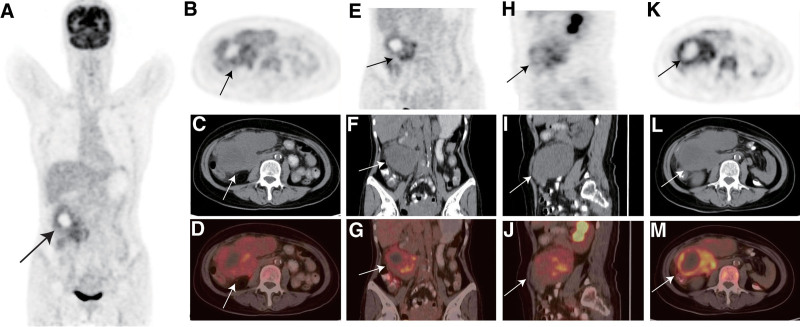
Maximum intensity projection image (A) showing a large irregular abnormal activity in the right abdomen (Black arrow). Axial (B: PET, C: CT, D: fused PET/CT), coronal (E: PET, F: CT, G: fused PET/CT) and sagittal (H: PET, I: CT, J: fused PET/CT) images show a right midupper abdominal mass measuring approximately 12.0 cm × 6.7 cm with a standardized uptake value maximum (SUVmax) of 5.4 on the first scan and a further increase in radioactive uptake with a SUVmax of 7.7 on the delayed scan (K: PET, L: CT, M: fused PET/CT). CT = computed tomography, PET = positron emission tomography, SUV = standardized uptake value.
